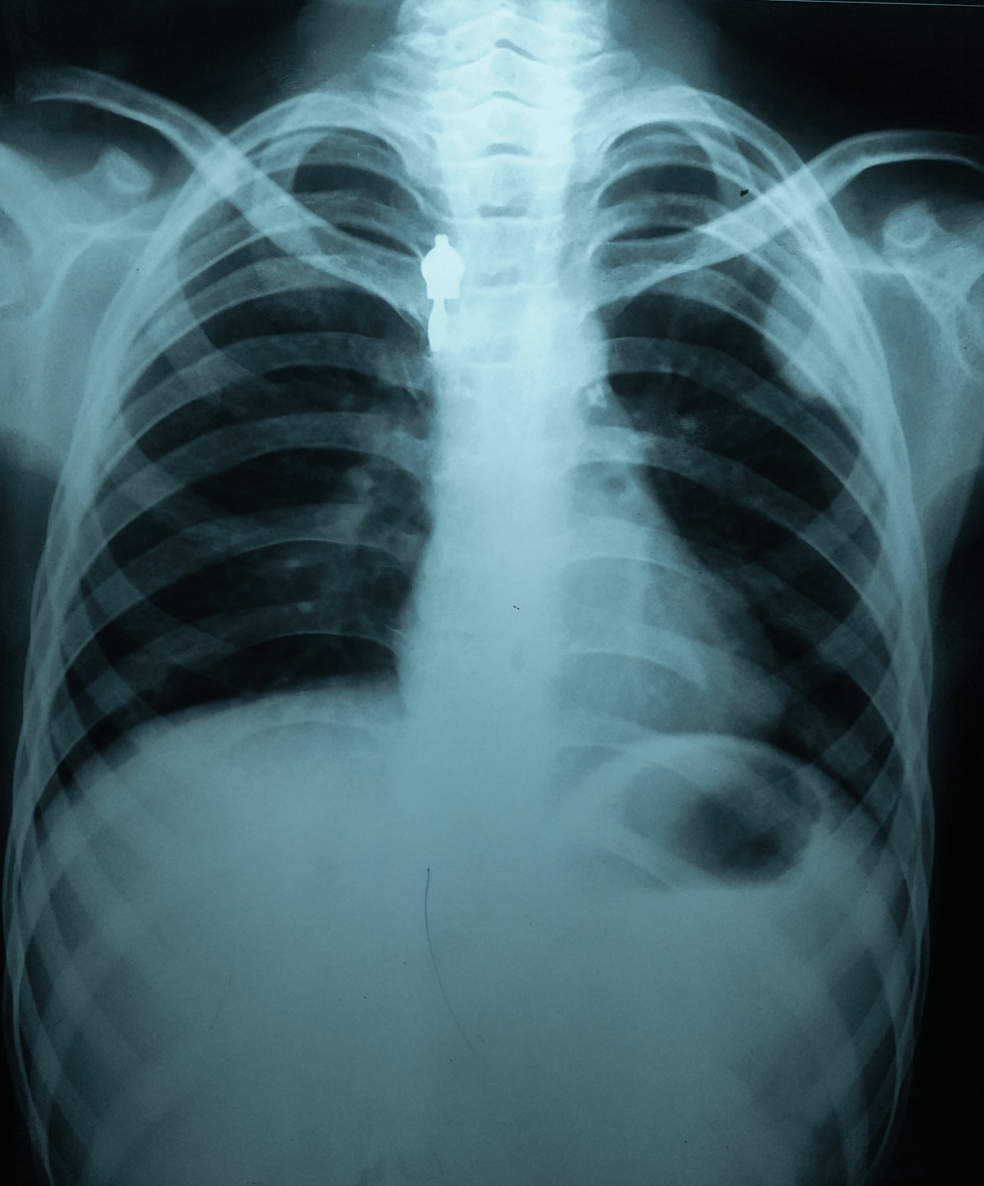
A chest radiograph (P-A) view not suggestive of any pulmonary disease P-A: postero-anterior
